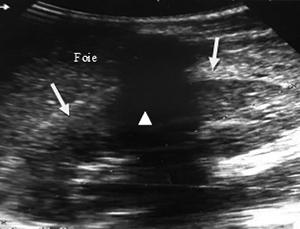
Ultrasound image of a small hematoma under the right kidney capsule. Hyperechoic border of liver and kidney (white arrow), arcuate measuring 11.6 mm thick.
